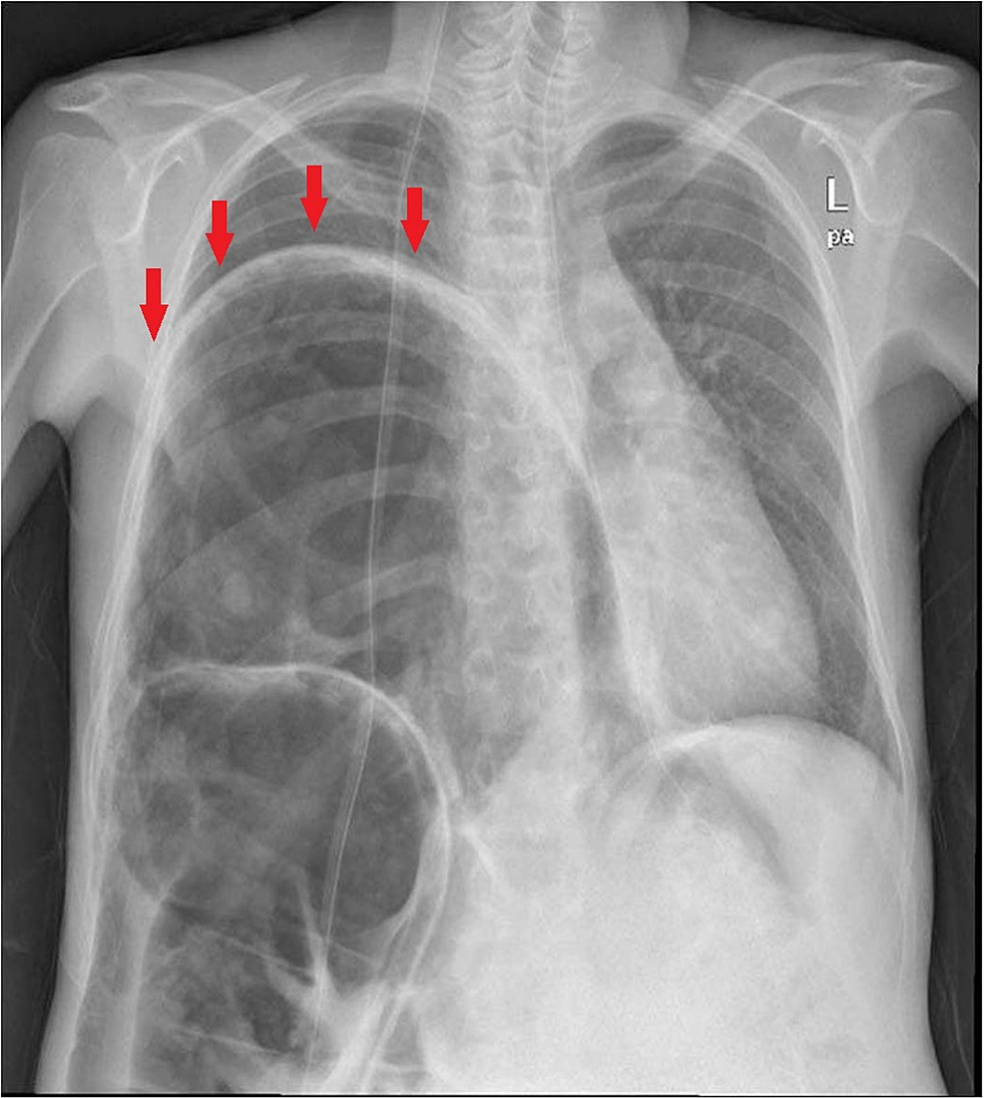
Chest radiography shows an excessive right diaphragmatic eventration and a distended bowel filled with gases under the right hemidiaphragm (red arrows).
